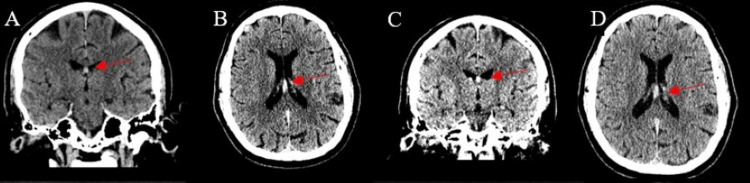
Non-contrast head CT performed during hospitalization A: Initial non-contrast head CT coronal slice demonstrating fornix hyperdensity (arrow). B: Initial non-contrast head CT axial slice with bilateral fornix hyperdensity (arrow). C: Non-contrast CT coronal slice (24 hours) unchanged fornix hyperdensity (arrow). D: Head CT axial slice (24 hours) with stable fornix hyperdensity (arrow). CT: computed tomography
